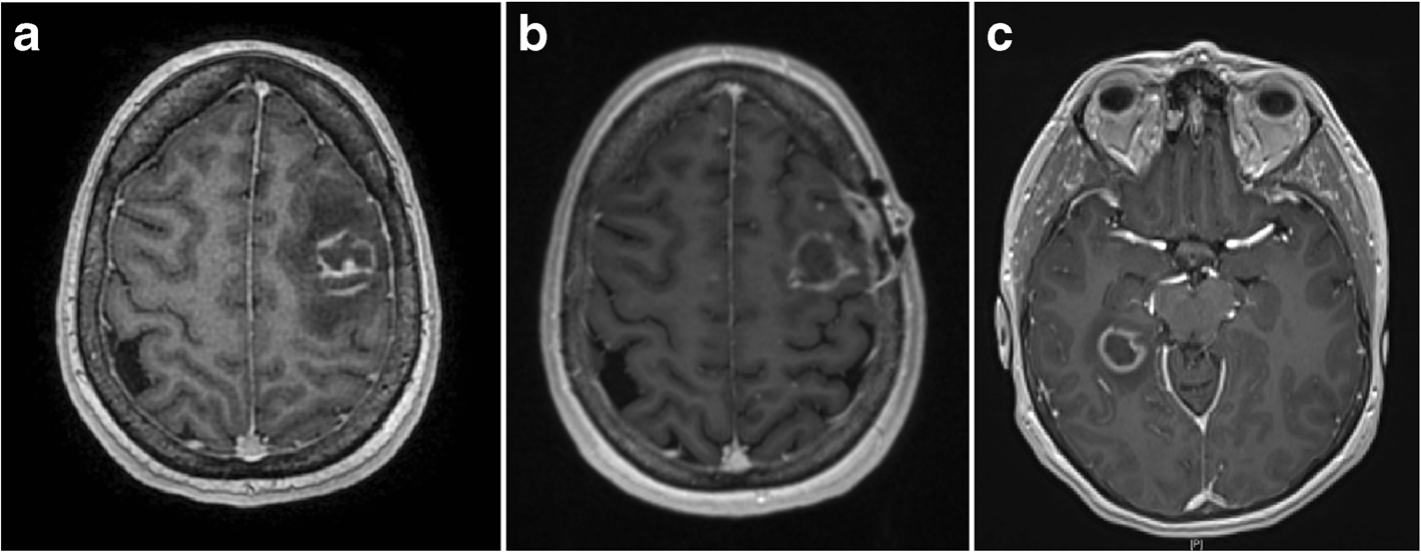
Progressive cerebral toxoplasmosis on interval neuroimaging. Serial magnetic resonance imaging (MRI) of the brain using sagittal T1 weighted images. At initial presentation there was (a) a solitary rim-enhancing lesion in the left posterior frontal lobe with moderate surrounding vasogenic odema and a 3.5 cm midline shift to the right. The patient had 4 weeks of therapy with oral sulfadiazine and pyrimethamine. Follow-up imaging showed (b) a persistent lesion in the left posterior frontal lobe as well as new miliary lesions in the cerebral hemispheres bilaterally and involvement of central grey structures including the right lentiform nucleus and thalamus. These findings were most consistent with disseminated toxoplasmosis. Four and a half months after the initial presentation and 4 weeks after a reduction to maintenance therapy the patient represented with nausea, headache and loss of consciousness and imaging showed (c) a new ring enhancing lesion in the right temporal lobe and persistent diffuse military nodules
